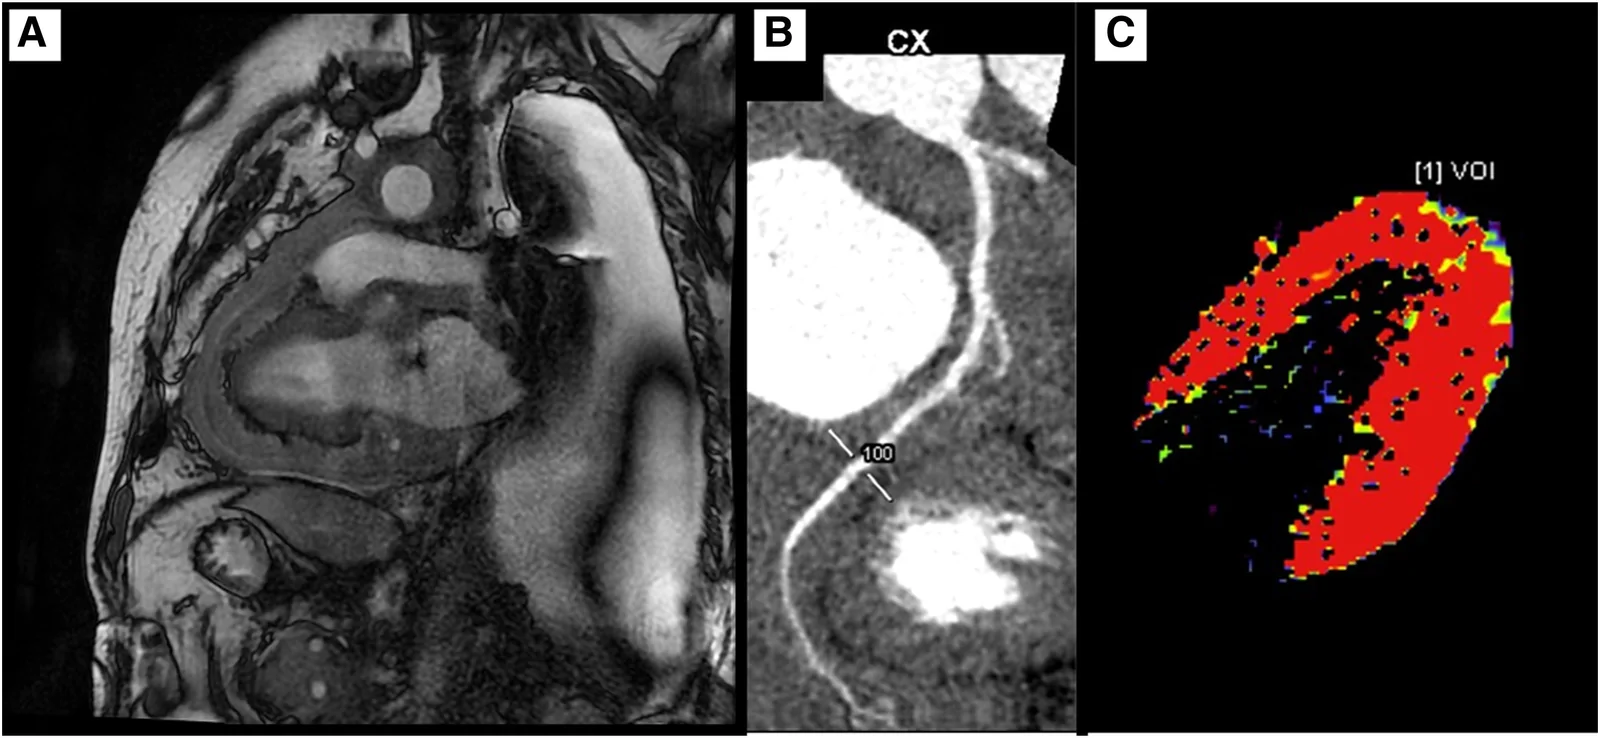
Cardiovascular magnetic resonance, computed tomography angiography, and myocardial perfusion imaging. Cardiovascular magnetic resonance demonstrated marked thickening of the pericardial layers, measuring up to 9–10 mm per layer, with pronounced post-contrast enhancement and a residual fluid layer of up to 4.6 mm between the pericardial layers (*A*). No significant coronary artery stenosis was identified on computed tomography angiography (*B*). No mismatch between myocardial blood flow (MBF) and myocardial blood volume (MBV) was observed (*C*).
